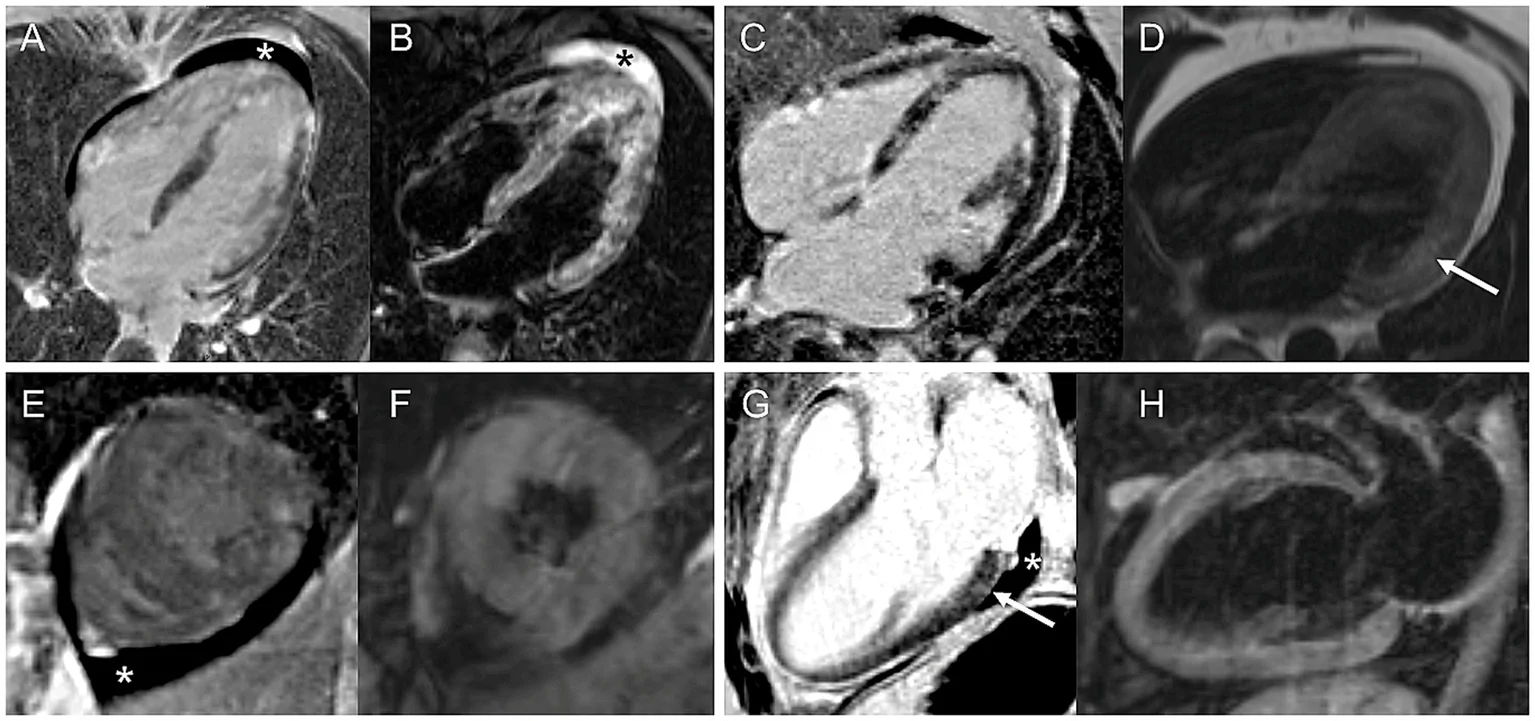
Cardiac magnetic resonance findings in acute EM across eosinophil-associated disorders. Upper left panels: Four-chamber phase-sensitive inversion recovery (PSIR) image (A) of a patient with eosinophilic granulomatosis with polyangiitis demonstrates patchy subendocardial, mid-myocardial, and subepicardial late gadolinium enhancement (LGE) involving both ventricles. Corresponding myocardial edema is evident on the T2-weighted image (B), with an associated pericardial effusion (asterisk). Upper right panels: Cardiac magnetic resonance (CMR) in a patient with hypereosinophilic syndrome demonstrates biventricular LGE with subendocardial predominance (C). Myocardial edema is particularly evident in the basal lateral wall of the left ventricle (D, arrow). Lower left panels: Short-axis CMR images of a patient with hypersensitivity/cephalosporin-associated EM demonstrate patchy subendocardial, mid-myocardial, and subepicardial LGE in the left ventricle, with predominant subendocardial involvement of the right ventricle (E) and semi-circumferential pericardial effusion on PSIR imaging (asterisk). The T2-weighted image (F) reveals diffuse myocardial edema with anterior wall predominance. Lower right panels: CMR imaging of a patient with idiopathic EM. The three-chamber PSIR view (G) demonstrates subtle subepicardial LGE in the basal inferolateral left ventricular wall (arrow) with adjacent small pericardial effusion (asterisk). Despite respiratory motion artifacts, the two-chamber T2-weighted image (H) is suggestive of patchy myocardial edema.
